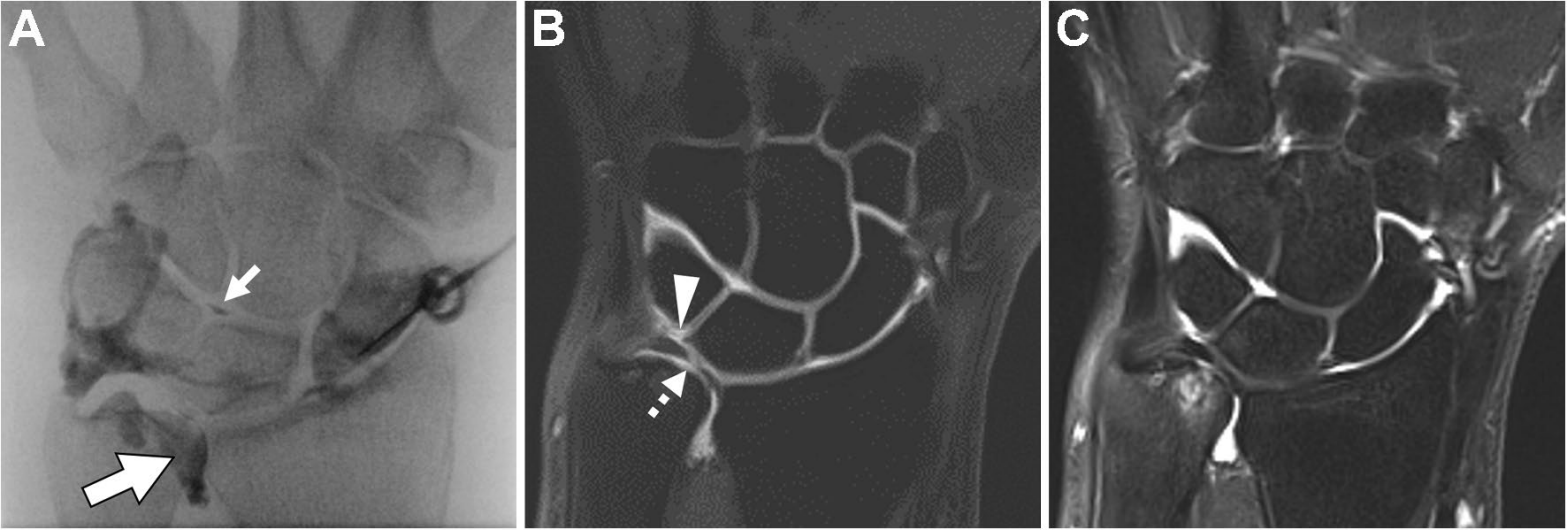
Suspected internal derangement of the wrist. Fifty-five-year-old man with wrist pain. A Spot image of the wrist after radiocarpal joint injection shows flow of contrast into the midcarpal (small arrow) and distal radioulnar (big arrow) joints. Coronal T1-weighted 1.5 T MR arthrogram image (B) shows a tear of the lunotriquetral interosseous ligament (arrowhead) and triangular fibrocartilage (dashed arrow), which were not as clearly shown on the coronal T2-weighted fat-suppressed image (C)
